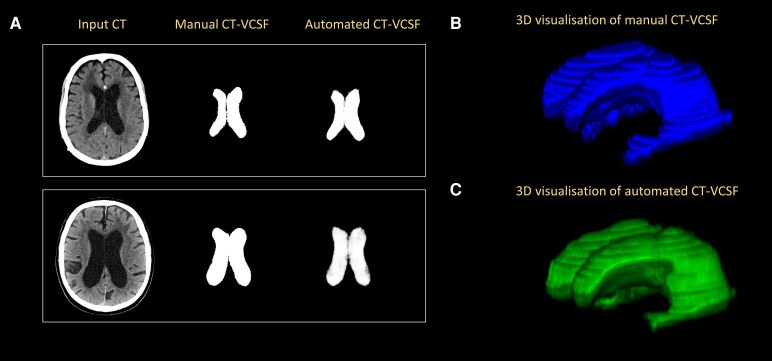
Model outputs. (A) Input CT images, manual lateral VCSF, and U-Net predicted lateral VCSF maps of two representative test iNPH patient datasets; (B) and (C) 3D visualization of manually derived and automatically derived CT-VCSF. Abbreviations: CT, computed tomography; lateral ventricular cerebrospinal fluid, VCSF.
